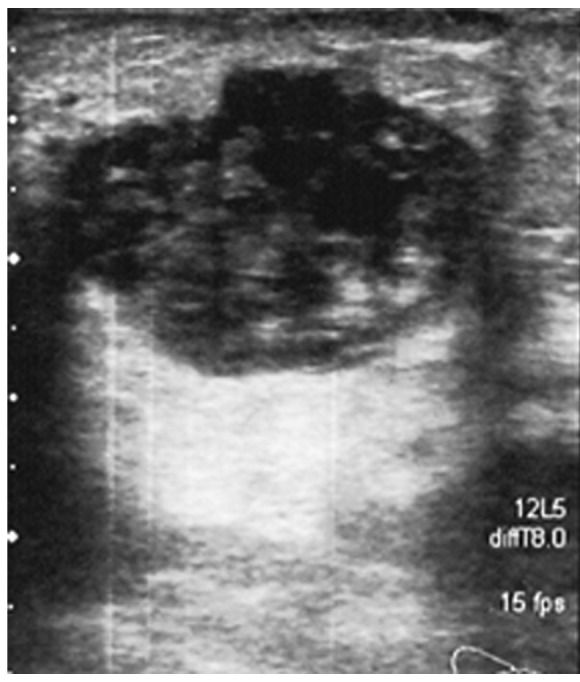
Ultrasonography of the tumor mass. The image reveals the presence of a hypoechoic solid neoformation with jagged edges and a maximum diameter of ~3 cm.
